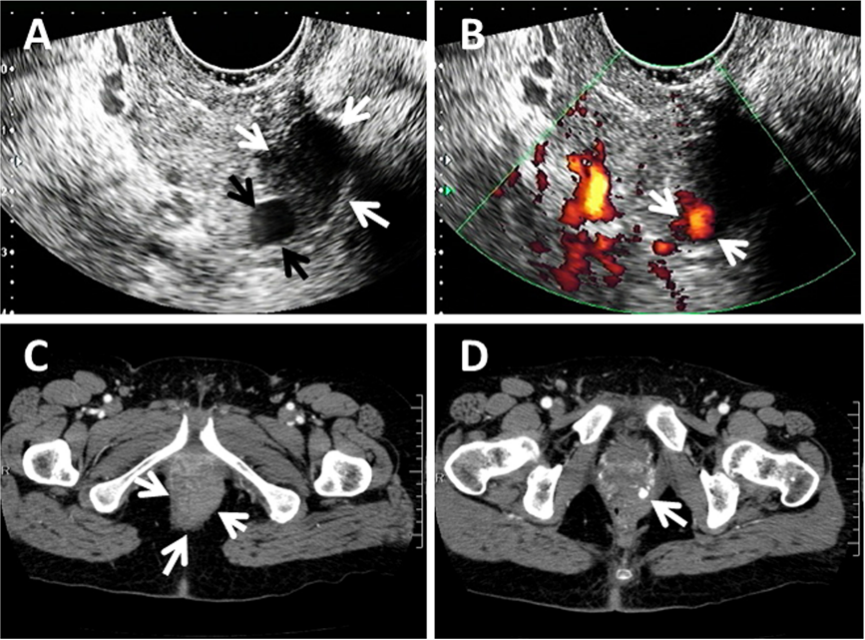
Transvaginal ultrasonography and pelvic contrast-enhanced computed tomography on the day of delivery. (A) pseudoaneurysm (black arrows) and adjacent hematoma (white arrows) in B-mode (B) pseudoaneurysm in power-Doppler mode. (C) hematoma (white arrow) on computed tomography (D) pseudoaneurysm (white arrows) on the next caudal slice of the hematoma.
